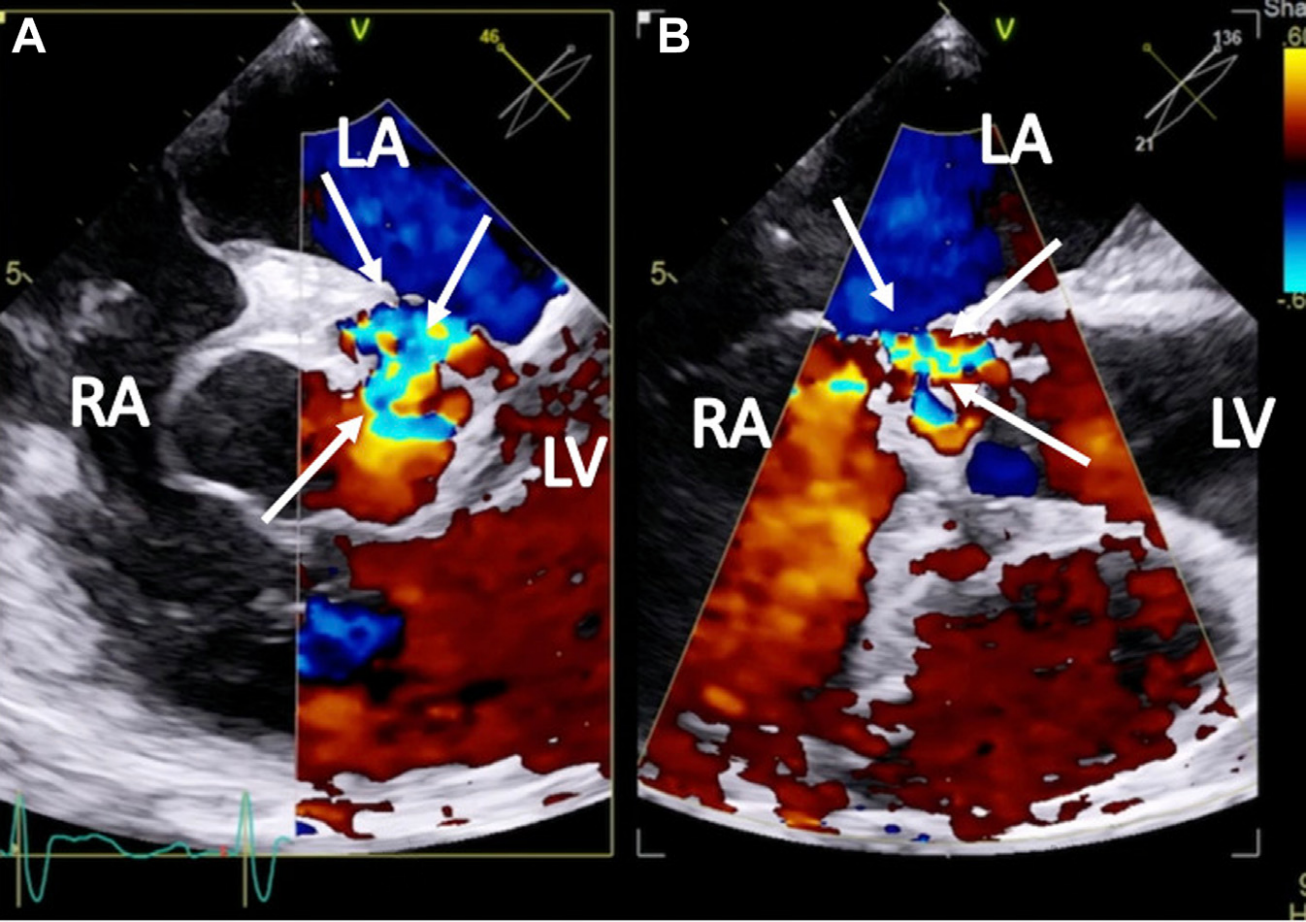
Transesophageal Echocardiographic Biplane Image at the Aortic Valve Level With Color Flow Doppler During Diastole (A) Short-axis view. (B) Long-axis view. The arrows demonstrate severe, anteriorly directed aortic regurgitation. LA = left atrium; LV = left ventricle; RA = right atrium.
